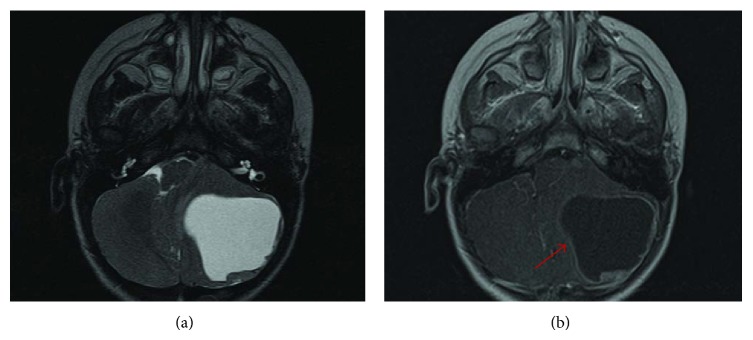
Axial T2 (a) and T1-contrast-enhanced (b) MR images of brain. There is a 5.6 × 5.1 cm largely cystic mass with peripheral nodular enhancement (arrow in (b)) involving the left cerebellar hemisphere. There is mass effect with compression of the 4th ventricle and effacement of the left premedullary cistern.
